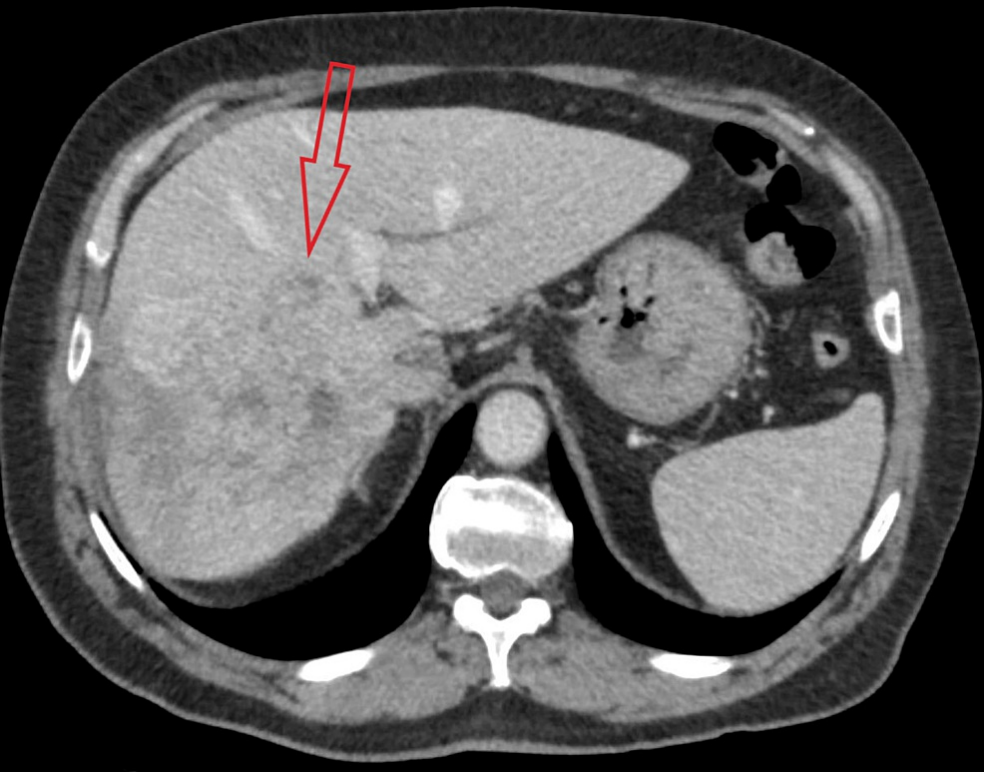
CT image of a tumor in the axial plane in the venous phase (the red arrow points to the right liver lobe tumor) CT: computed tomography
